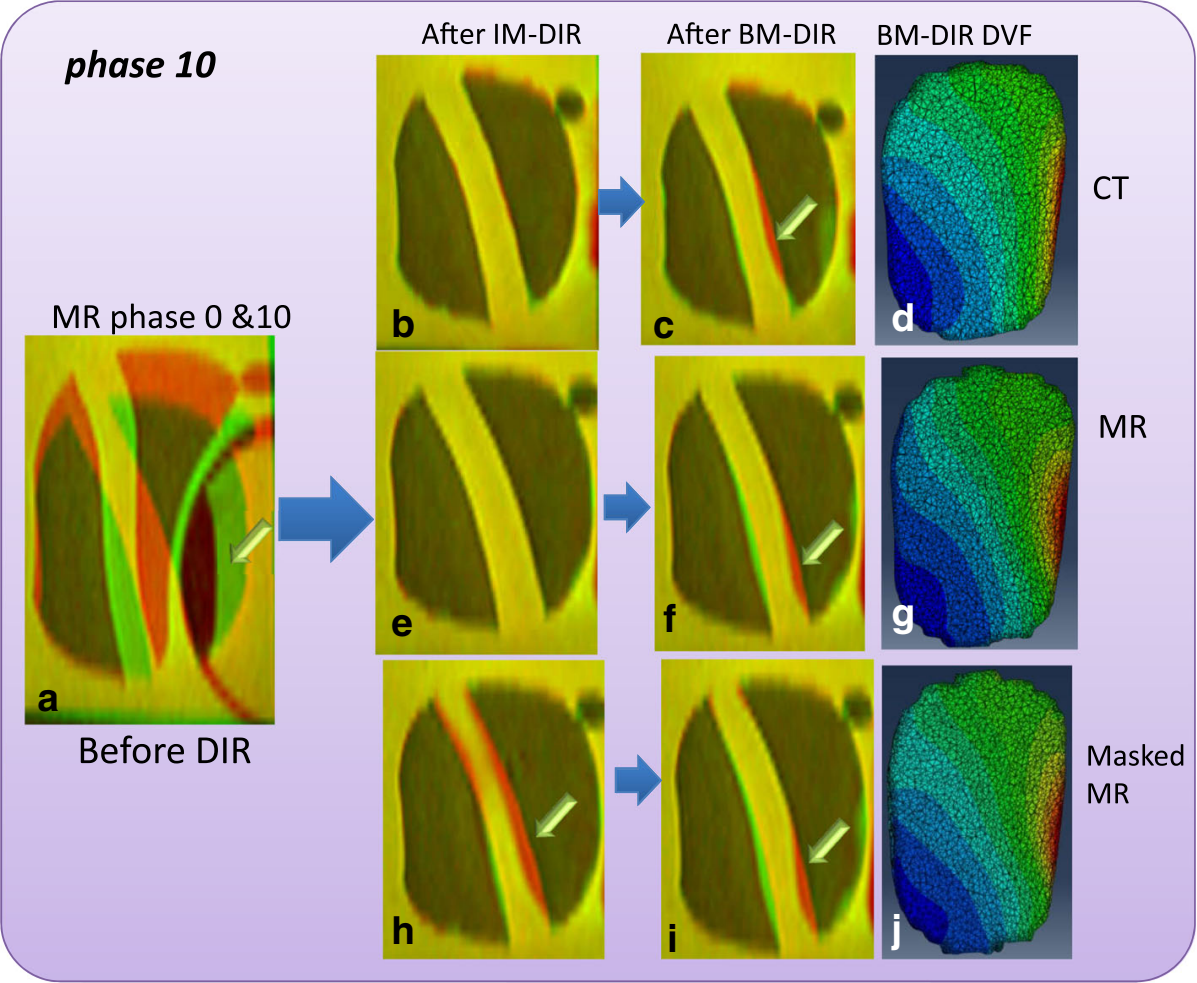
Image fusion for DIR between Phase 0 & 10: a before DIR; IM-DIR with (b) CT, e MR, h Masked MR; BM-DIR with (c) CT, f MR, i Masked MR; color rendering motion magnitude of (d) CT, g MR, j Masked MR. The green arrow points to sub-optimal matching of urethra structure after DIR
